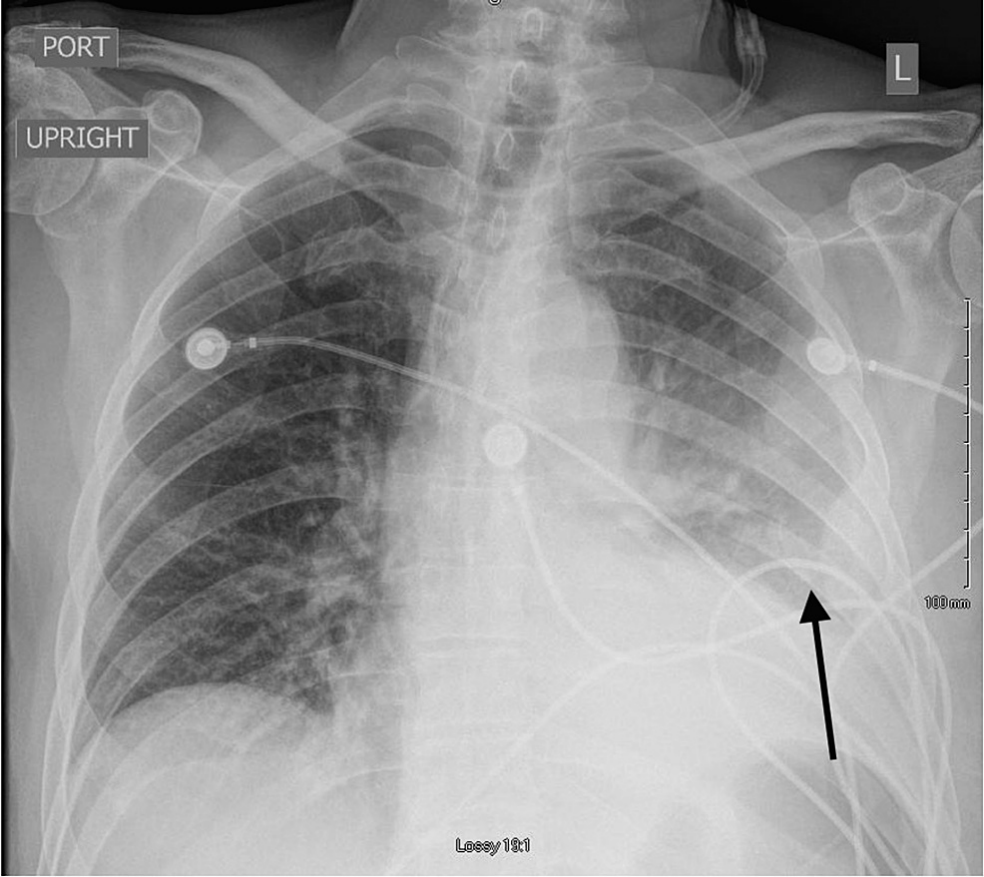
Follow-up Chest X-Ray A follow-up chest x-ray showing the progression of the left-sided opacity (arrow).
